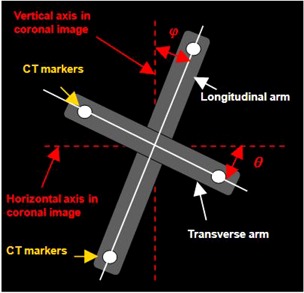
A pictorial representation shows the definition of ϕ and θ from the coronal view of volumetric jig CT scan. ϕ is the acute angle formed by the longitudinal arm of the jig and the longitudinal axis of the image, and θ is the acute angle formed by the transverse arm of the jig and the transverse axis of the image. The angel will be positive if the arm is in clockwise direction with respect to the axis; otherwise, it will be negative.
